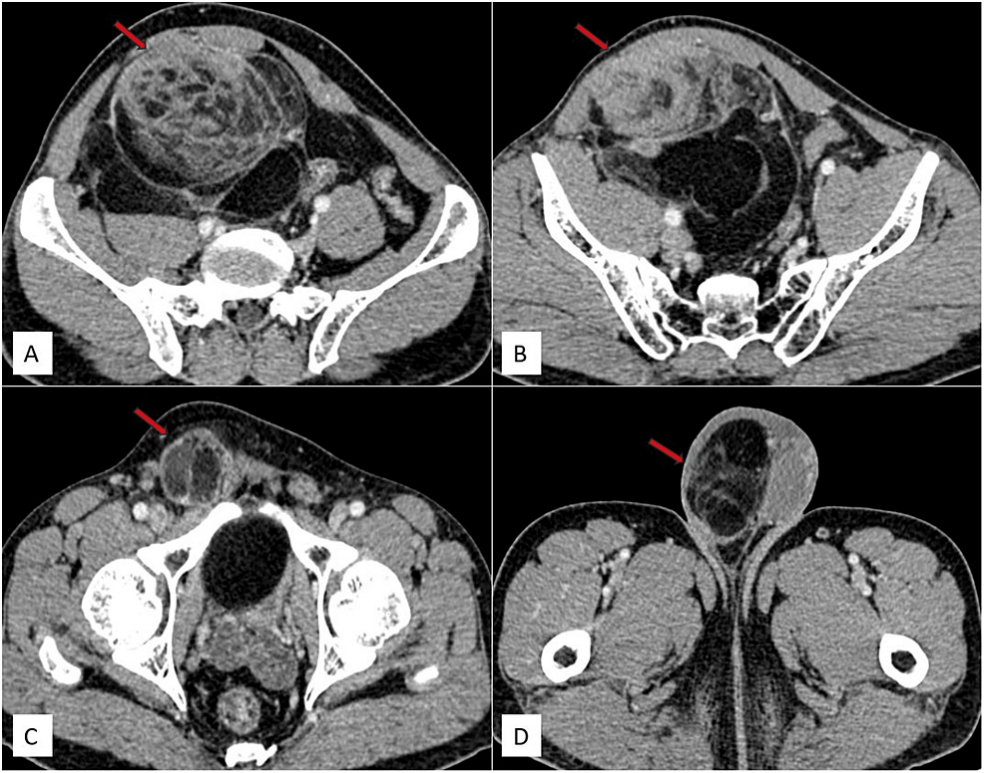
Axial contrast CT images depict a solid mass of heterogeneous density, showing both lipomatous and non-lipomatous components, in the retroperitoneum. It extends inferiorly into the pelvis, right inguinal region, and right scrotal sac up to the upper pole of the right testis. The solid component exhibits heterogeneous enhancement. Axial contrast CT images reveal a mass of heterogeneous density in the retroperitoneum (red arrow) (A), extending inferiorly into the pelvis (red arrow) (B) and right inguinal region (red arrow) (C) and reaching the right scrotal sac up to the upper pole of the right testis (red arrow) (D). CT: computed tomography
